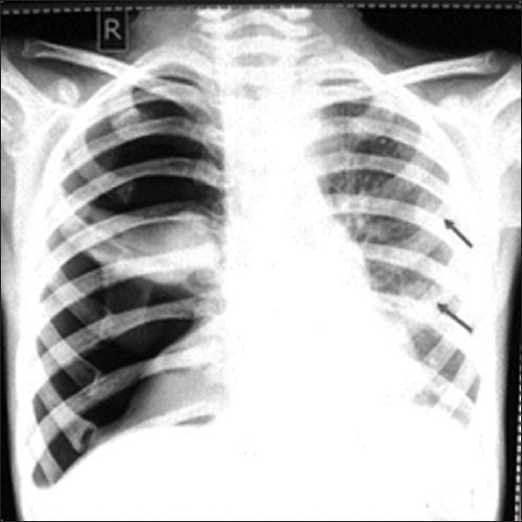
Chest radiograph showing right-sided tension pneumothorax and multiple cavitating nodules in left lung (arrows)
